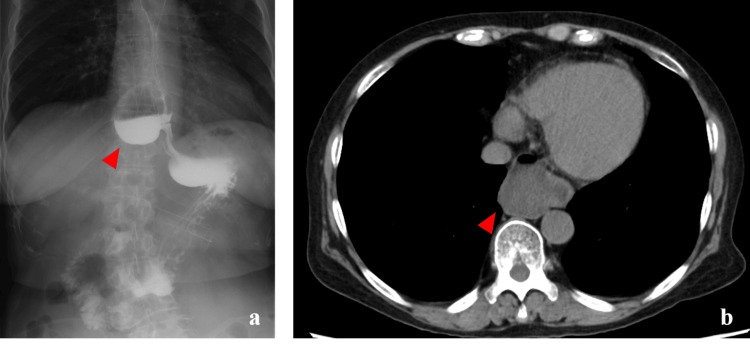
Preoperative imaging findings (a) Preoperative upper gastrointestinal imaging showing a diverticulum on the right side of the thoracic esophagus just above the diaphragm. Even after the contrast agent flowed into the stomach, residual contrast remained within the diverticulum (red arrow); (b) Preoperative CT image showing food residue remaining within the diverticulum on the right side of the esophagus (red arrow). CT: computed tomography
